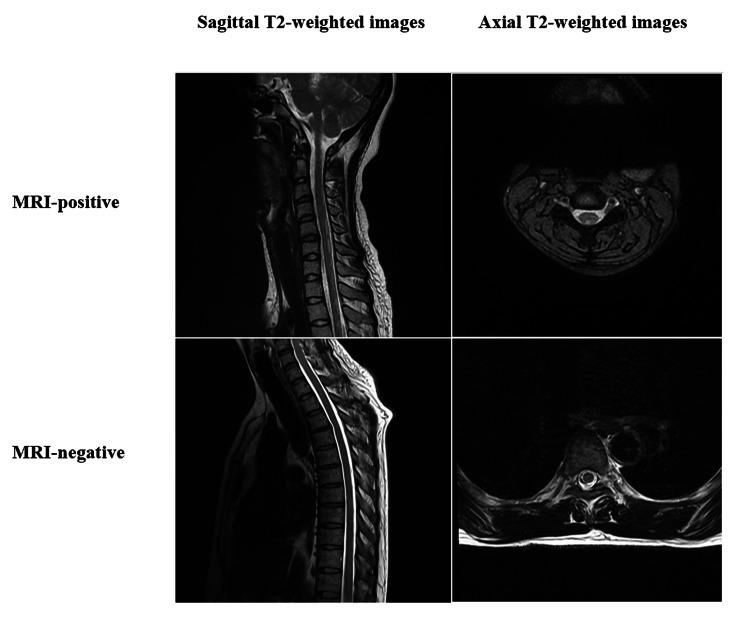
Sagittal/Axial T2-weighted images showing representative examples of spinal cord MRI of patients with MRI-negative and MRI-positive IATM.
